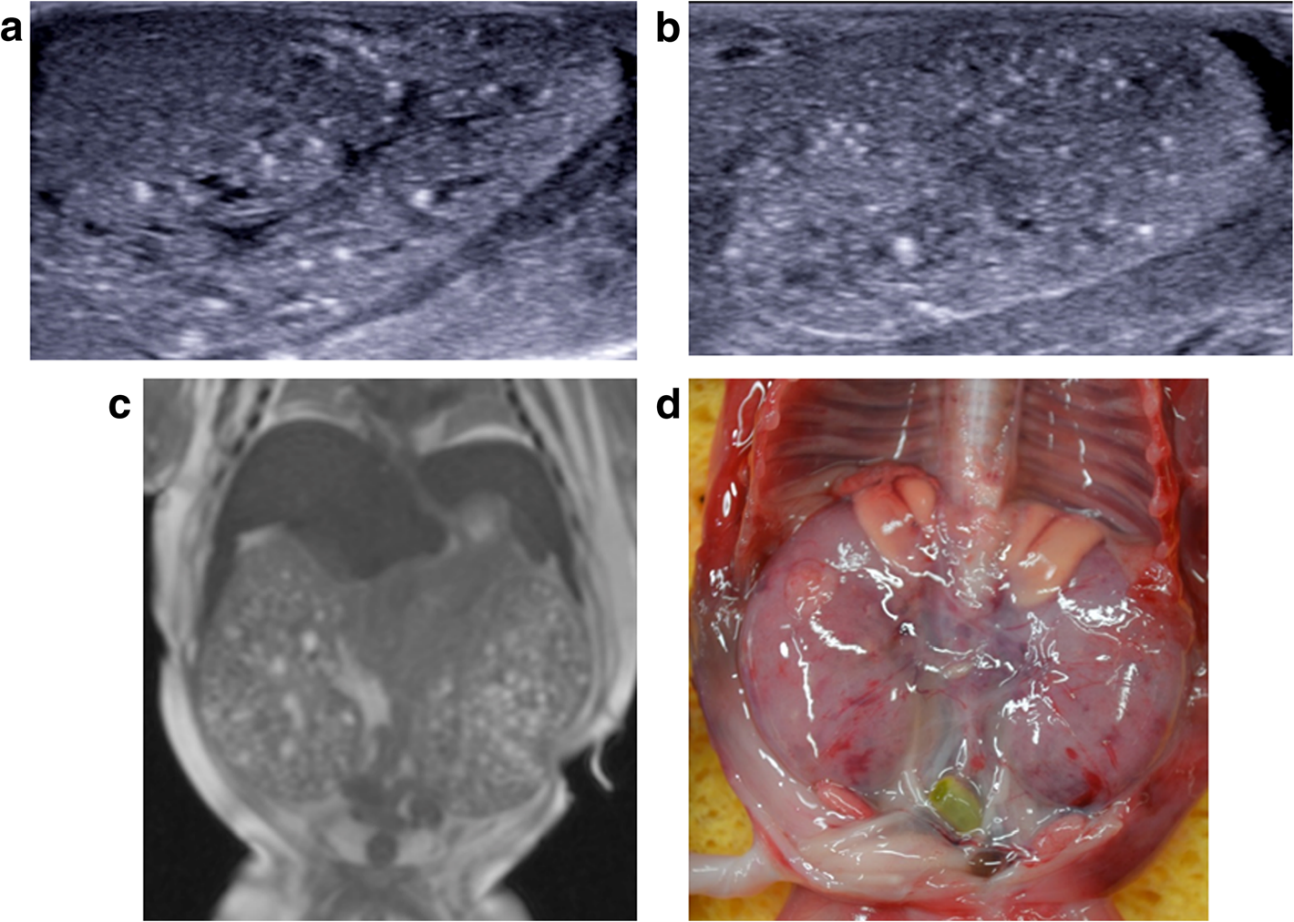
a–c Autosomal recessive polycystic kidney disease in a foetus at 17 weeks gestation, following termination of pregnancy. The post-mortem imaging was obtained 8 days after delivery. The sagittal post-mortem ultrasound images of the right (a) and left (b) kidneys demonstrate poor corticomedullary differentiation, numerous hyperechoic foci and a hyperechoic renal cortex in keeping with numerous renal microcysts. The corresponding post-mortem T2 weighted MRI in coronal plane (c), also shows enlarged kidneys with small microcysts and a normal appearing liver. At autopsy, the photograph taken of the retroperitoneum, with both kidneys in situ (d), shows the large size of these organs, occupying almost the entire abdomen
